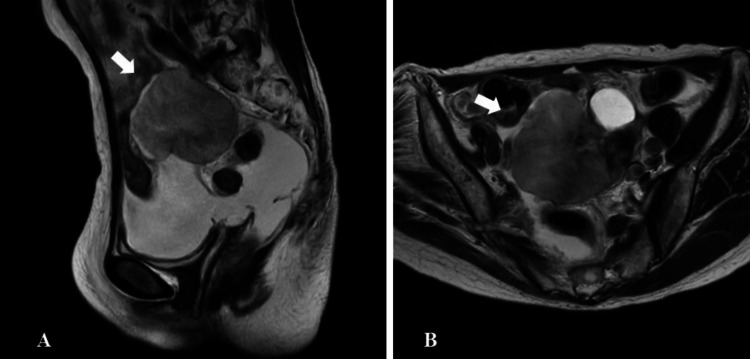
Magnetic resonance imaging (MRI) at the time of recurrence. (A) Sagittal T2-weighted MRI reveals a pelvic mass in 8 cm diameter (arrow) and ascites. (B) Axial T2-weighted MRI.
